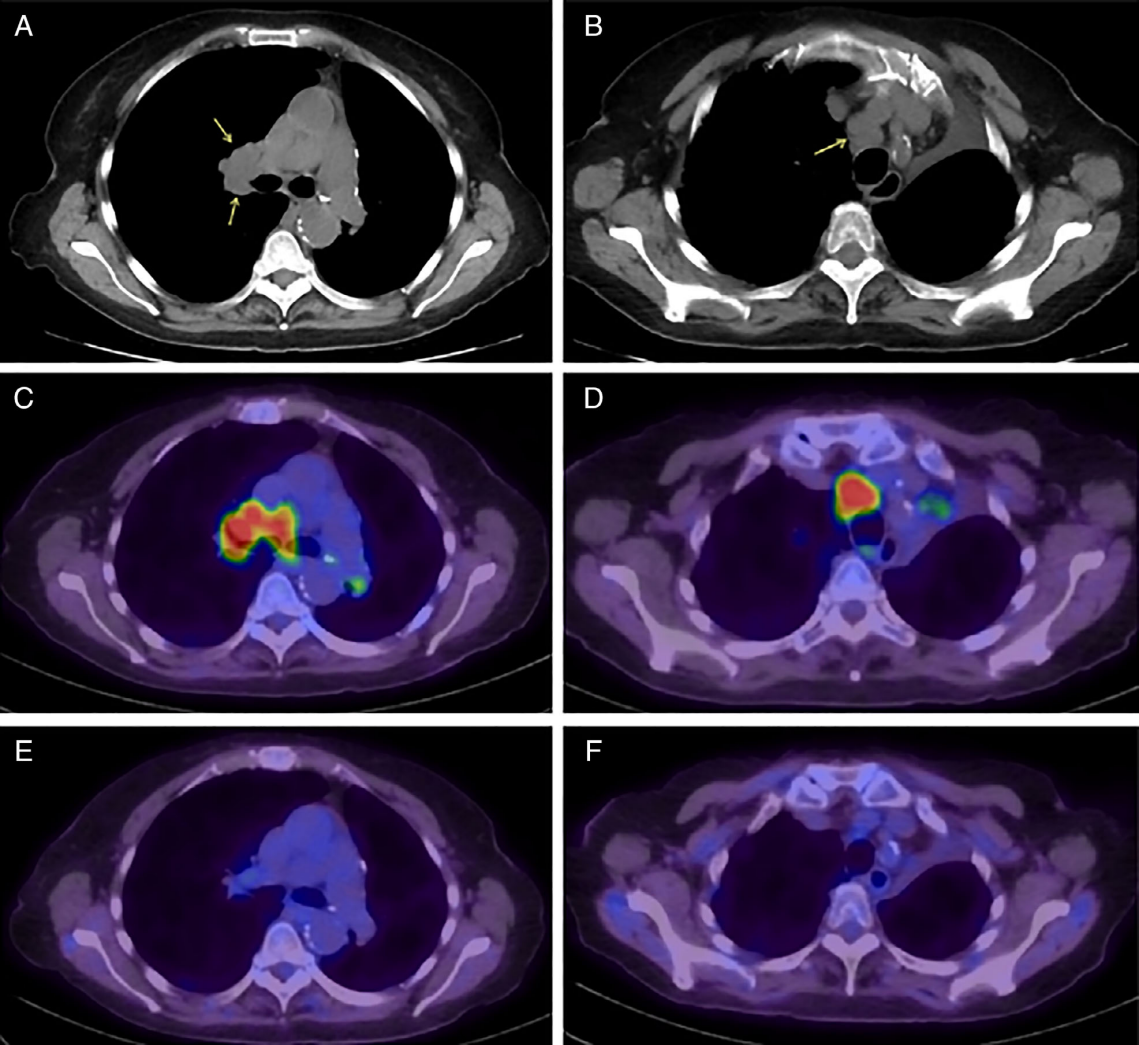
(A, B) Non‐contrast computed tomography (CT) of the chest with bilateral mediastinal lymphadenopathy involving the right pre‐tracheal nodal station, left pre‐vascular space, and subcarina. (C, D) Positron emission tomography (PET)‐CT demonstrating highly metabolic active lymphadenopathy. (E, F) PET‐CT three months after the cessation of durvalumab demonstrating complete resolution of metabolically active bilateral hilar and mediastinal lymphadenopathy.
